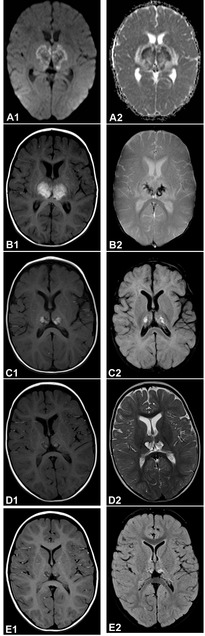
(A1) Diffusion-weighted image (DWI) revealed increased diffusion in the bilateral thalami and periventricular cerebral white matter. (A2) The apparent diffusion coefficient (ADC) map showed three different patterns of the thalamus and cerebral white matter. (B1) Axial T1-weighted images after 5 days showed symmetric bilateral hyperintensities located deep in the bilateral thalami (hemorrhage).(B2) Multi-planar gradient recalled (MPGR) axial images after 5 days showed symmetric bilateral hypointensities located deep in the bilateral thalami (hemorrhage). (C1) Axial T1-weighted images after 1 month showed a considerable regression of symmetric bilateral hyperintensities located deep in the bilateral thalami. (C2) Axial fluid attenuated inversion recovery (FLAIR) images after 1 month showed a considerable regression of symmetric bilateral hyperintensities located deep in the bilateral thalami. (D1) Axial T1-weighted images after 6 months showed a decrease in the size of symmetric bilateral hyperintensities located deep in the bilateral thalami. (D2) Axial T2 weighted images after 6 months showed a decrease in the size of symmetric bilateral hyperintensities located deep in the bilateral thalami. (E1) Axial T1-weighted images after 2 years showed a considerable decrease in the size of symmetric bilateral hyperintensities located deep in the bilateral thalami. (E2) Axial FLAIR images after 2 years showed a considerable decrease in the size of symmetric bilateral hyperintensities located deep in the bilateral thalami.
